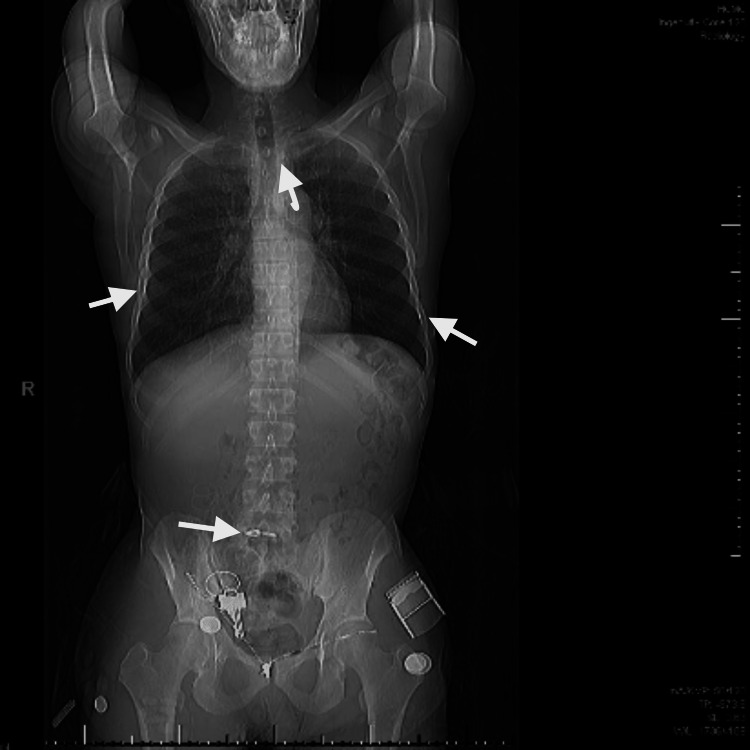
Scout scan of computed tomography imaging showing diffuse skeletal sclerosis
